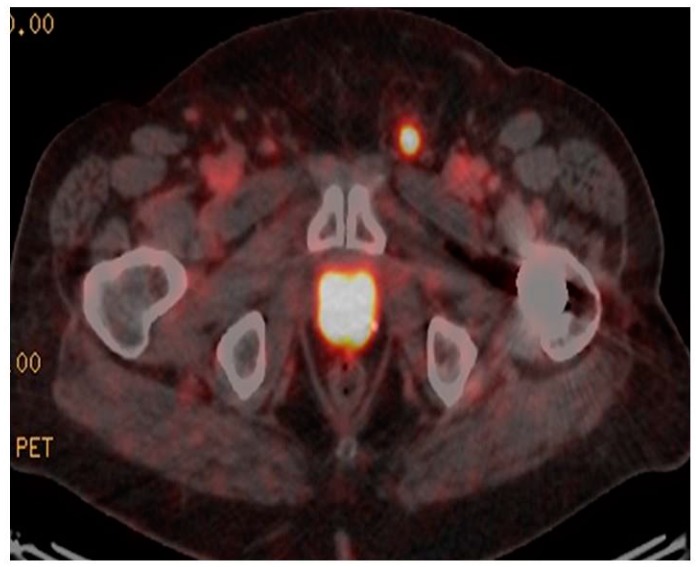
Staging ^68^Ga-PSMA PET/CT. Gleason 4 + 5. PSA 19 ng/mL. Intense ^68^Ga-PSMA avid primary disease in prostate with ^68^Ga-PSMA avid superficial left inguinal node metastasis.
